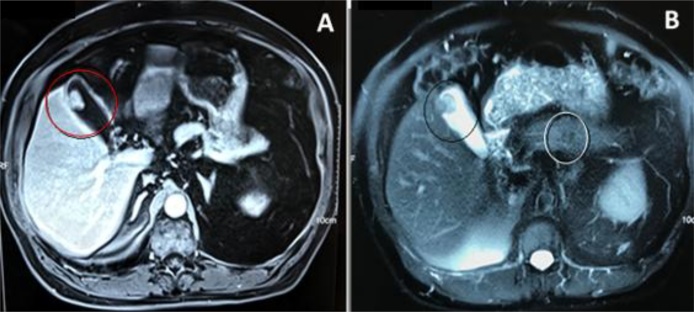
Abdominal MRI. A. Expansive formation on the right lateral body wall of the gallbladder, with 1.7 × 1.3 cm, showing pronounced early and persistent contrast enhancement and promoting exophytic bulging of the underlying outer vesicular margin, which shows irregular contours (Red circle). B.T2-weighted hypointense expansive formation in the right lateral body wall of the gallbladder (black circle) and T2-weighted slightly hyperintense nodular formation in the body portion of the pancreas (White circle).
